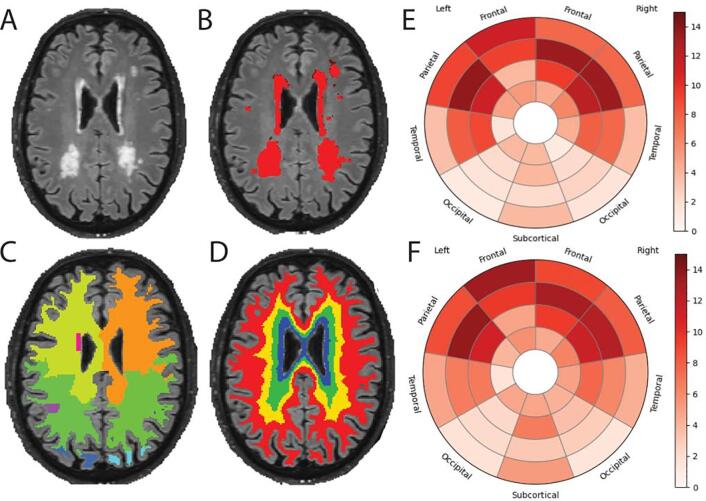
FLAIR derived phenotypes. A) Example FLAIR scan from one EPAD participant with relatively high lesion volume; B) Result of the white matter hyperintensities (WMH) segmentation using BaMoS; C, D) Lobes and layer atlases, respectively, used for regional WMH volume computation, methodological details are given in (Sudre et al., 2018); E, F) Effect of age on WMH frequency (expressed in percentage of increase in frequency, i.e. the proportion of lesion in a given region, per additional year of age) for male and female respectively.
